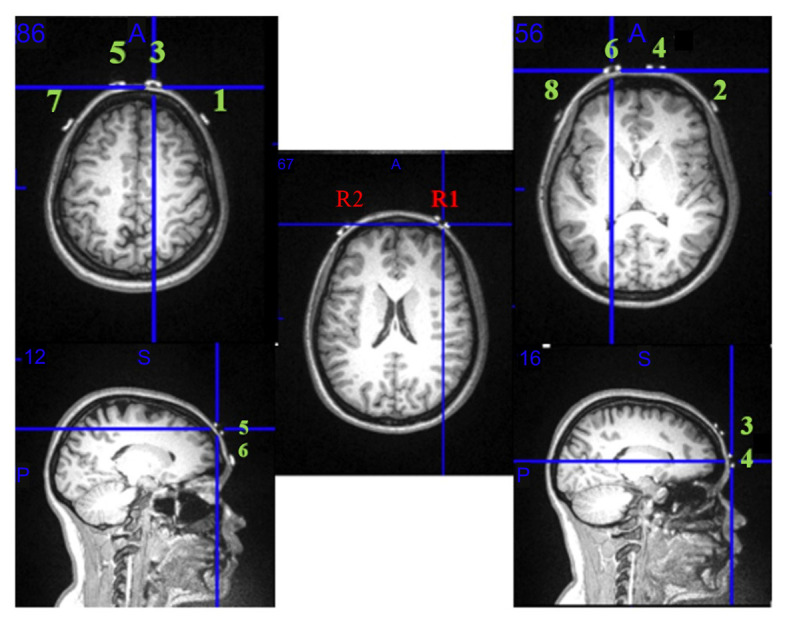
fNIRS optode configuration mapped onto an MRI scan. MRI demonstrates that all the optodes and receivers on the fNIRS are positioned over the right and left Brodmann area 10. R1 and R2 represent detectors; the green numbers 1 through 8 are the fNIRS infrared light emitters.
